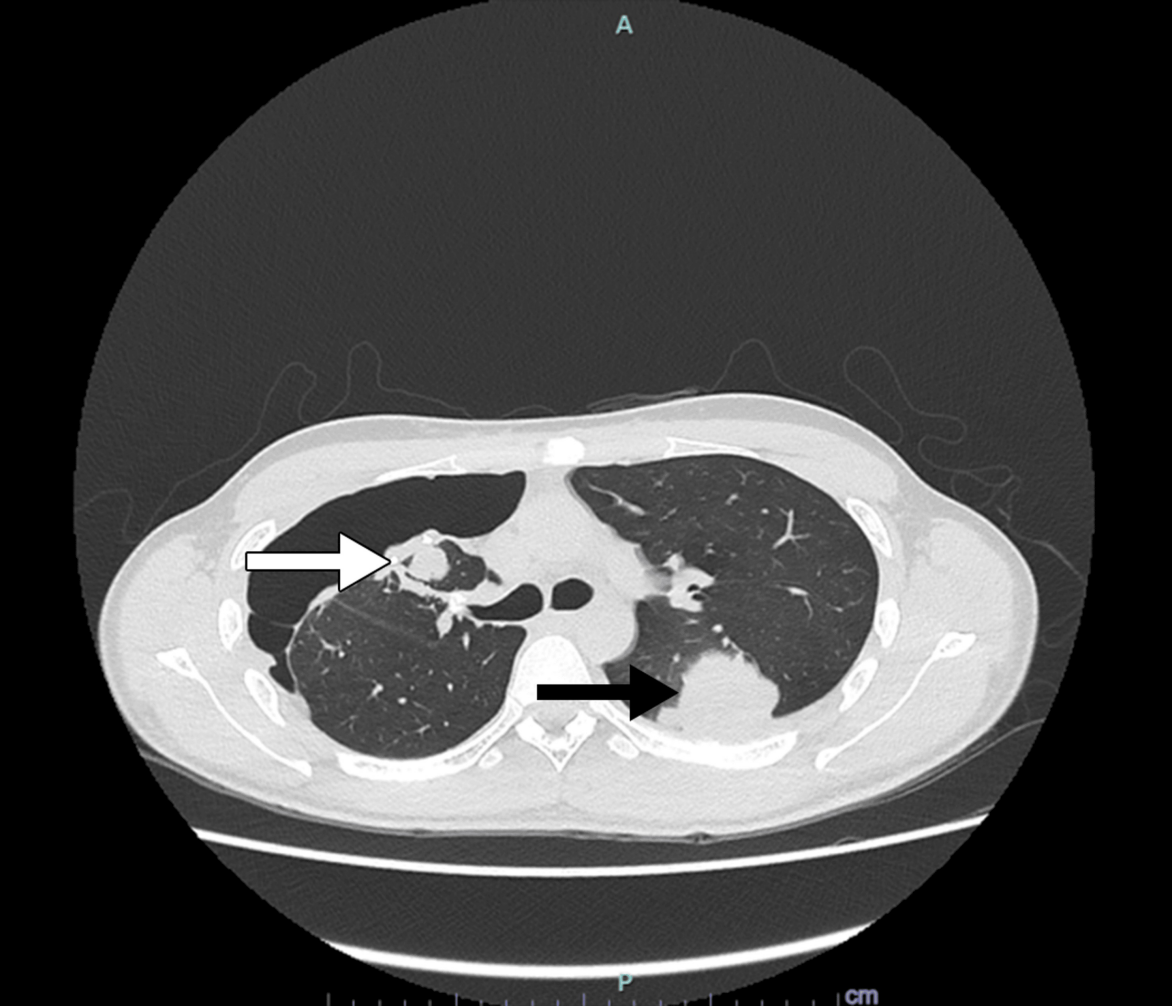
An axial view of the CT thorax showing lung nodules in the left lower lobe (black arrow) as well as the collapsed right upper lobe (white arrow) with right pneumothorax.
